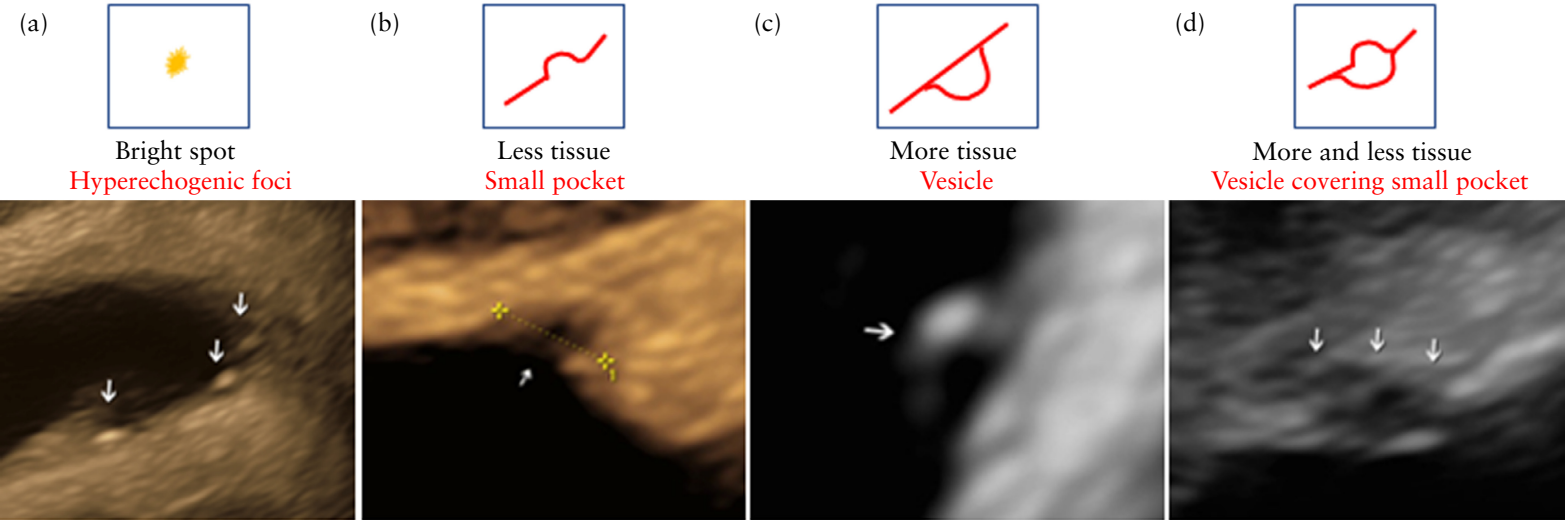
Summary of ultrasonographic findings associated with the presence of superficial endometriosis. Findings may appear in isolation (a,b,c) or in combination (d), and different lesions may show different associated findings within the same patient.
